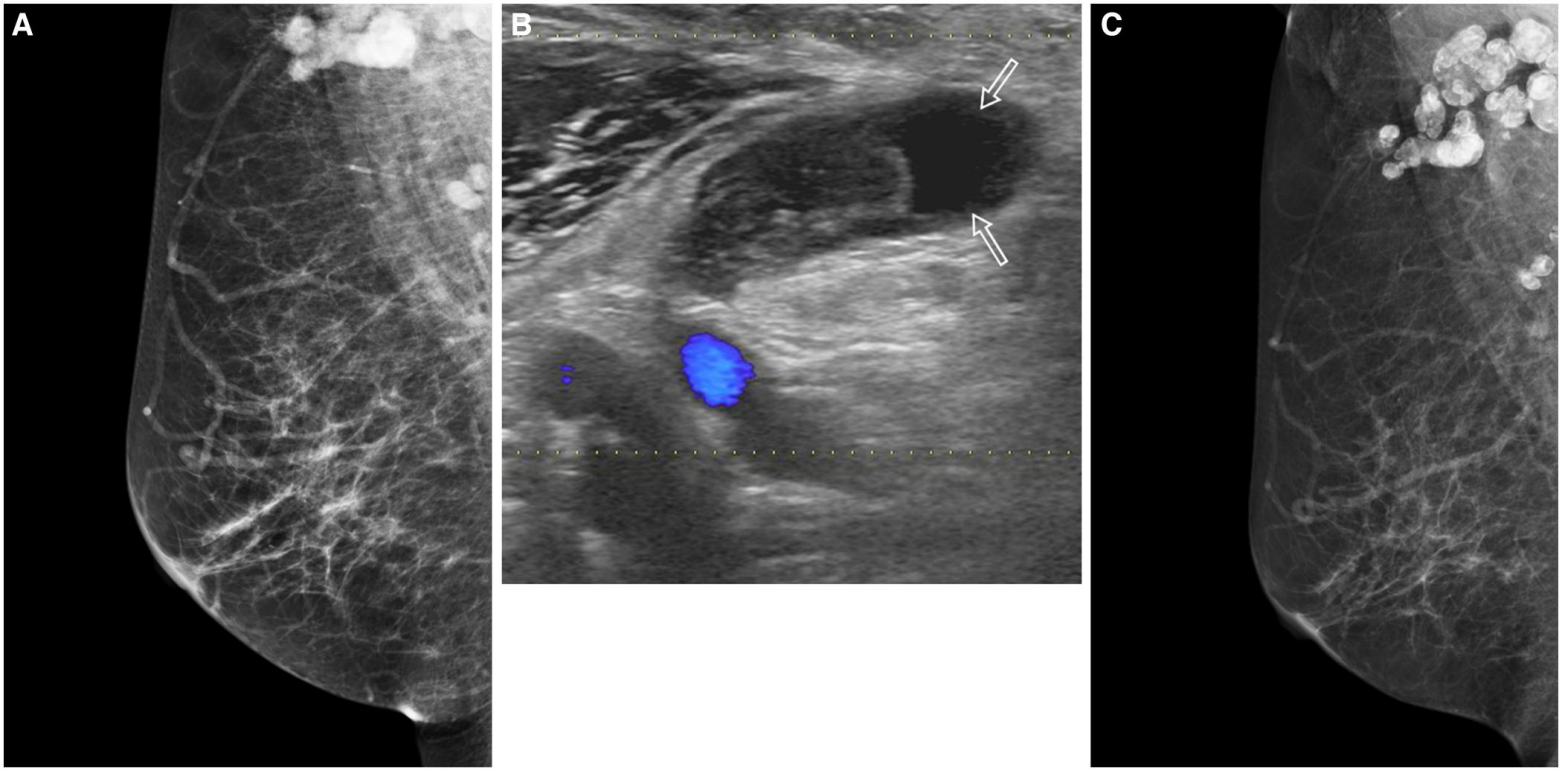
Tuberculous (TB) lymphadenitis in a 56-year-old woman who presented with a palpable right axillary mass. (A) Right medial-lateral oblique (MLO) mammogram image shows multiple enlarged, dense axillary lymph nodes without abnormality in the breast. (B) Corresponding colour Doppler ultrasound (US) image shows an enlarged axillary lymph node with heterogeneous echogenicity and internal cystic component (arrows). US-guided fine-needle aspiration of the internal cystic component showed caseous necrotic material with positive acid-fast bacilli. (C) Right MLO mammogram taken 1 year following anti-TB therapy shows coarse calcifications in the enlarged axillary lymph nodes.
